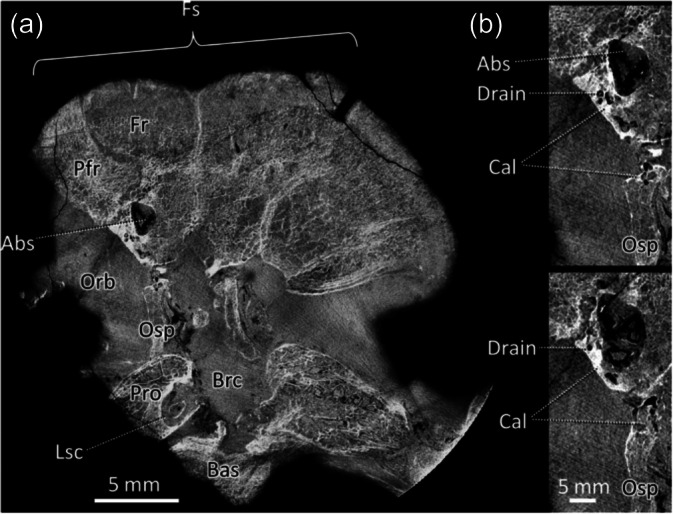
CT virtual cross‐section through the braincase of *Moschognathus whaitsi* (AM 4950). Abscess marked as Abs. (From Benoit et al., 2026). Scale bars equal 5 mm.
